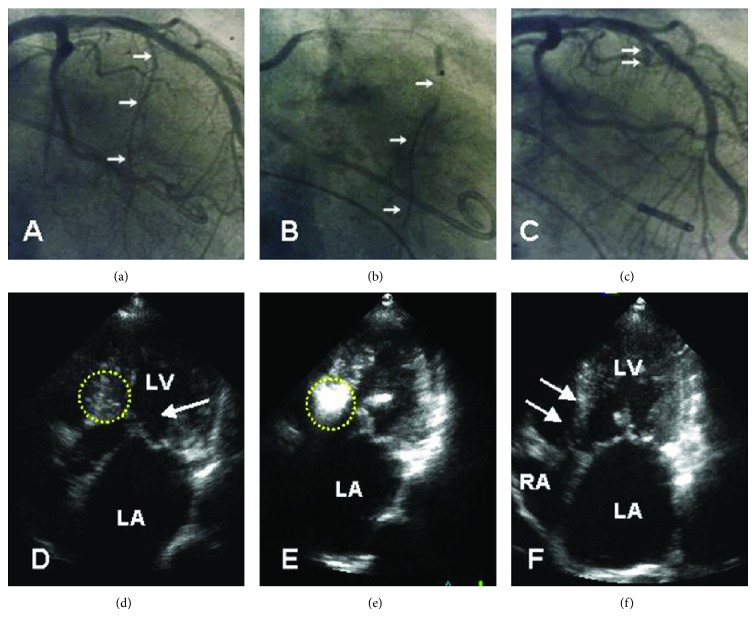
Angiographic ((a)–(c)) and echocardiographic ((d)–(f)) aspect of an echotargeted septal ablation procedure (in our practice denominated as PTSMA). A guidewire is advanced into the target vessel (arrows in (a)). Subsequently, an over-the-wire balloon is introduced. The correct position and fit of the balloon are verified by contrast injection (arrows in (b)) through the central catheter lumen. The vessel stump after alcohol injection and removal of the balloon is shown in (c) (arrows). In (d), the dotted circle marks the septal target area including the SAM-septal contact zone. Contrast injection into the target vessel (e) precisely highlights this area. After 3–6 months, akinesia and thinning of the subaortic septum are clearly visible, comparable to a myectomy trough.
